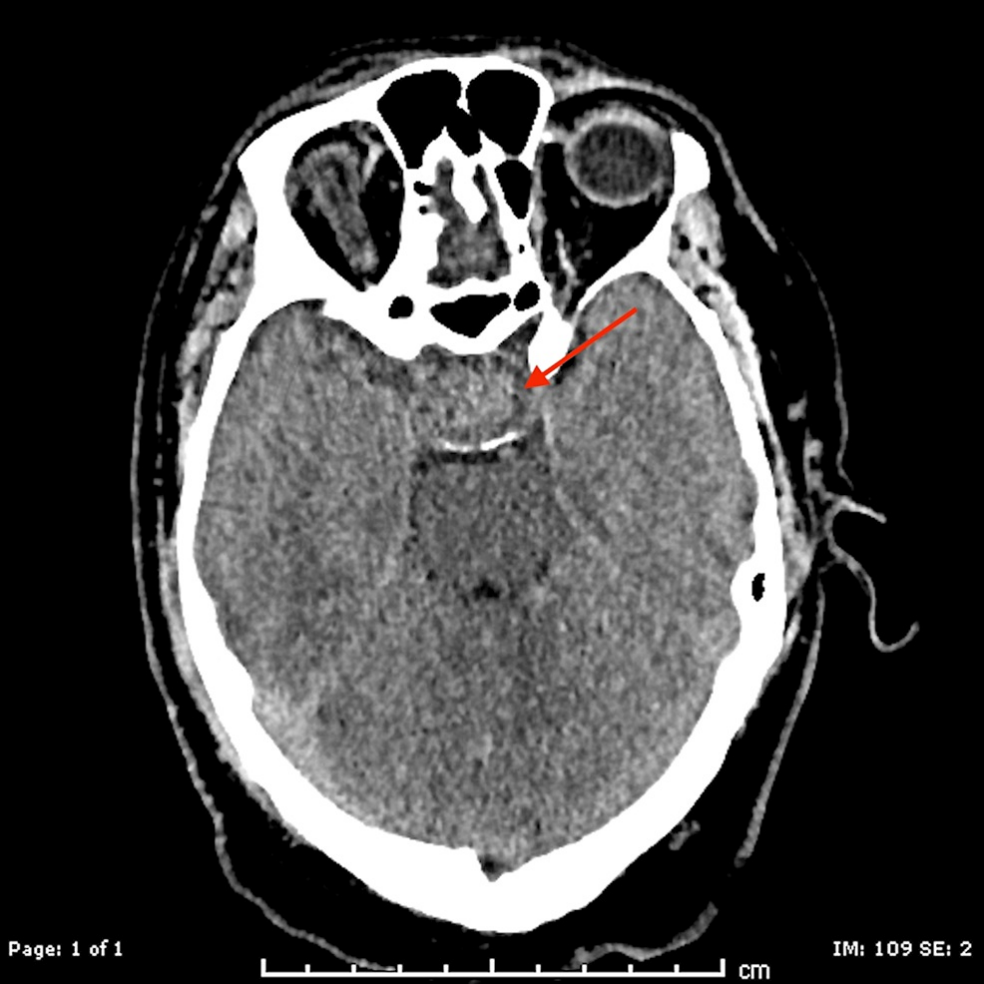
CT head taken after the initial development of new neurological findings on physical exam. The arrow highlights a heterogeneous soft tissue mass displaying characteristics consistent with pituitary macroadenoma. CT: computed tomography
